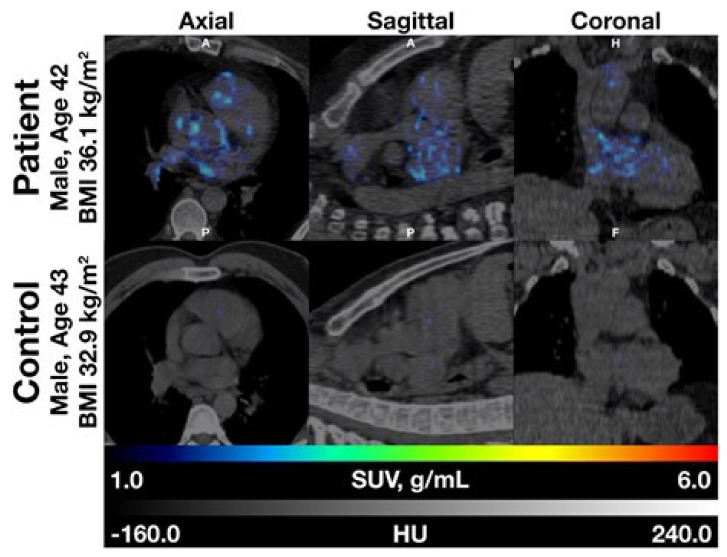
Fused NaF-positron emission tomography/computed tomography images of patient (top) and matched control (bottom). Note that the distribution of NaF uptake in the patient does not follow only the epicardial routes of the three coronary arteries, but is more dispersed, suggesting that NaF uptake is present in the walls of the entire cardiac arterial tree (with permission from reference [4]). Coronary arterial contrast-enhanced imaging would help address the issue.
